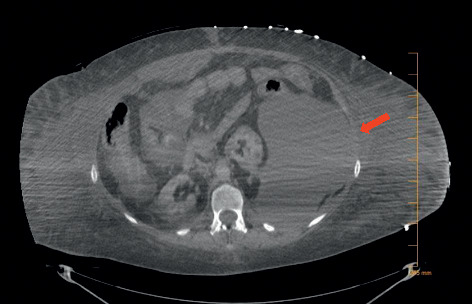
CT performed on day 9 showing development of a massive left-sided retroperitoneal hematoma.
